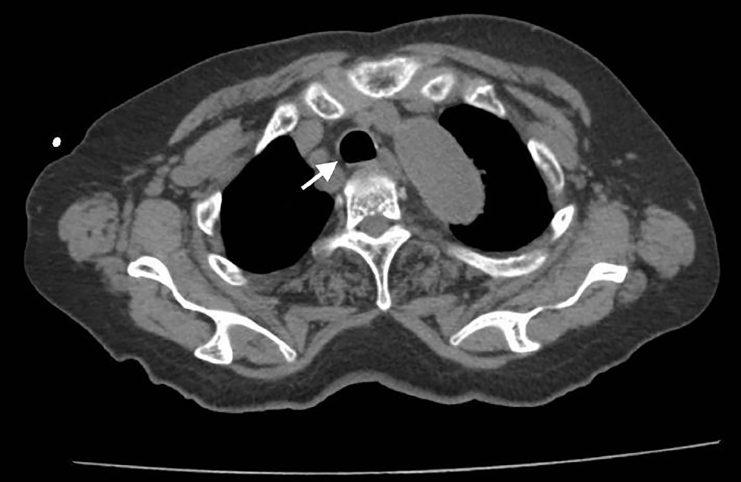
Repeated computed tomography with normal trachea (arrow).
